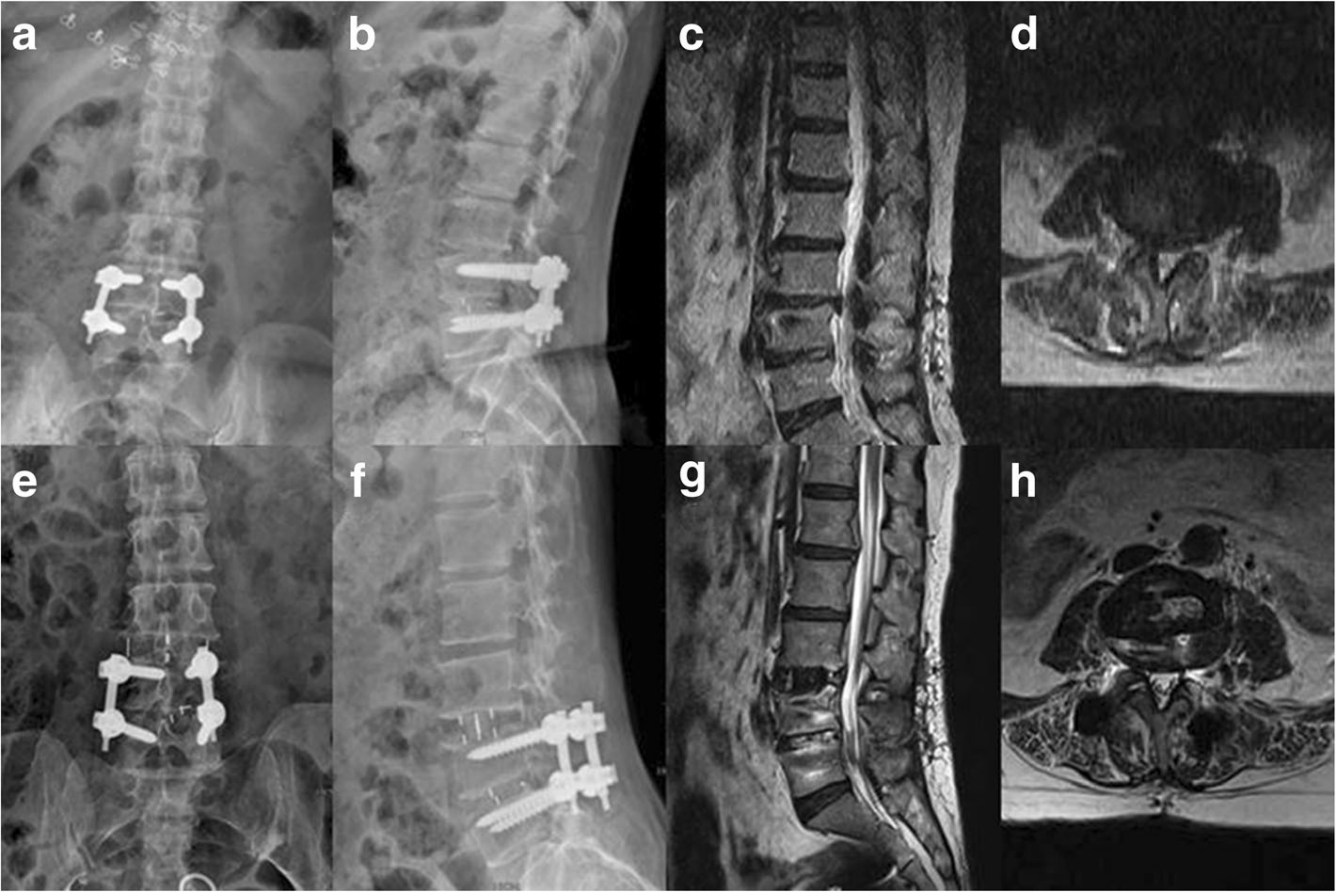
A 52-year-old female with a symptomatic ASD at the L3/4 level. Primary transforaminal lumbar interbody fusion was performed to treat the disc herniation at L4/5 2 years ago. OLIF without posterior instrumentation was employed to treat ASD at L3/4. a, b Preoperative X-ray shows loss of intervertebral disc height at L3/4, and posterior instrumentation and an intervertebral cage were placed appropriately at L4/5. Preoperatively, the IVH was 8.9 mm, the IFH was 15.4 mm, and the IFA was 1.76 cm^2^. c, d Preoperative MRI shows inclusive disc herniation at L3/4 and compression of right L4 nerve root. No obvious proliferation or calcification of the ligamentum flavum or zygopophysis was noted. Preoperatively, the APD was 6.6 mm and the CSA was 0.70 cm^2^. e, f Postoperative X-ray shows the intervertebral cage at L3/4 was well in place, and the IVH, IFH, and IFA were significantly increased. Postoperatively, the IVH was 14.8 mm, the IFH was 18.8 mm, and the IFA was 2.85 cm^2^. g, h Postoperative MRI shows the spinal canal was apparently enlarged and effective indirect decompression was achieved. The APD was improved from 6.6 mm preoperatively to 8.6 mm postoperatively, and the CSA postoperatively was 0.92 cm^2^, which was apparently larger than 0.70 cm^2^ preoperatively
